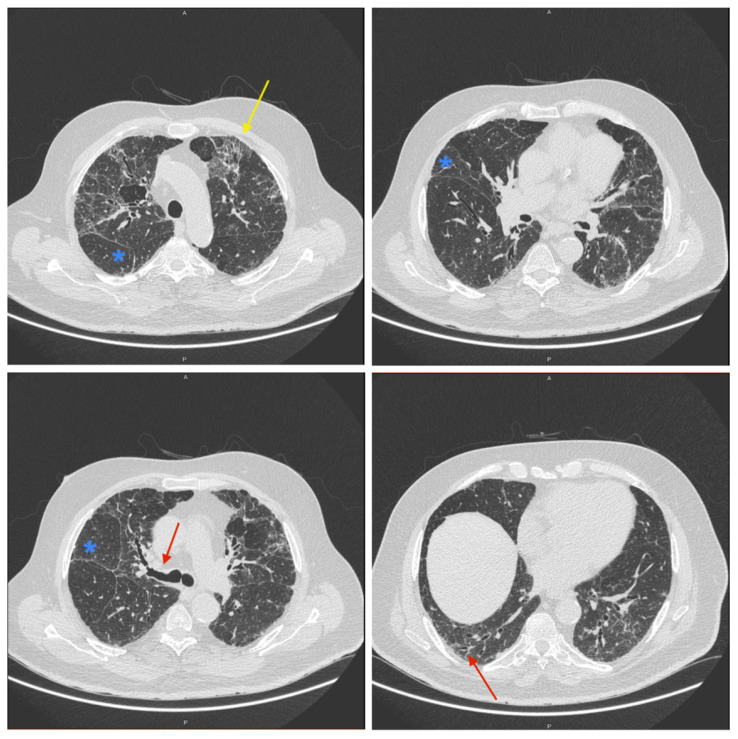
Chest high-resolution computed tomography (HRCT) performed at T0 showing traction bronchiectasis (red arrow), ground glass opacities (yellow arrow) and septal thickening (blue asterisk).
